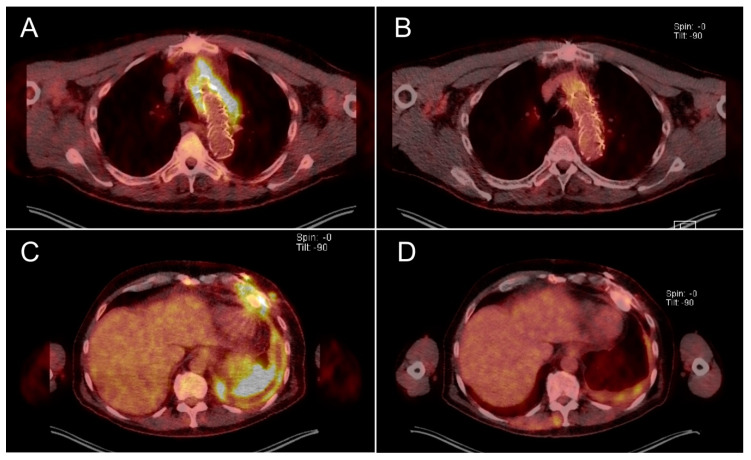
PET-CT scans of Patient 3 before (A) and seven months after (B) phage therapy in the area of the aortic graft and of Patient 4 before (C) and two months after (D) phage therapy in the area of the left ventricular assist device (LVAD) and pleural cavity empyema. Yellow emission shows level of accumulation of the tracer substance (2-[^18^F]fluoro-2-desoxy-D-glucose), which corresponds to inflammation.
